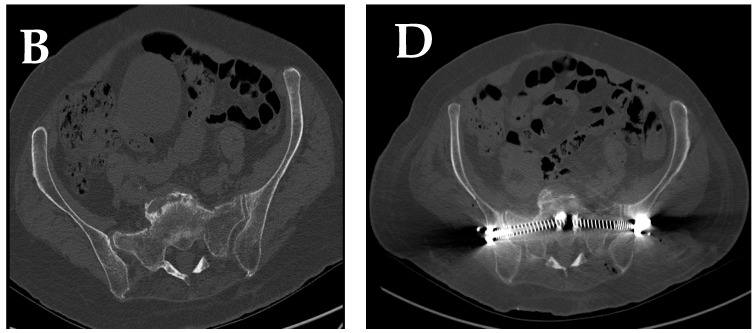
Preoperative (A,B) and postoperative (C,D) coronal and axial CT scan cuts.
